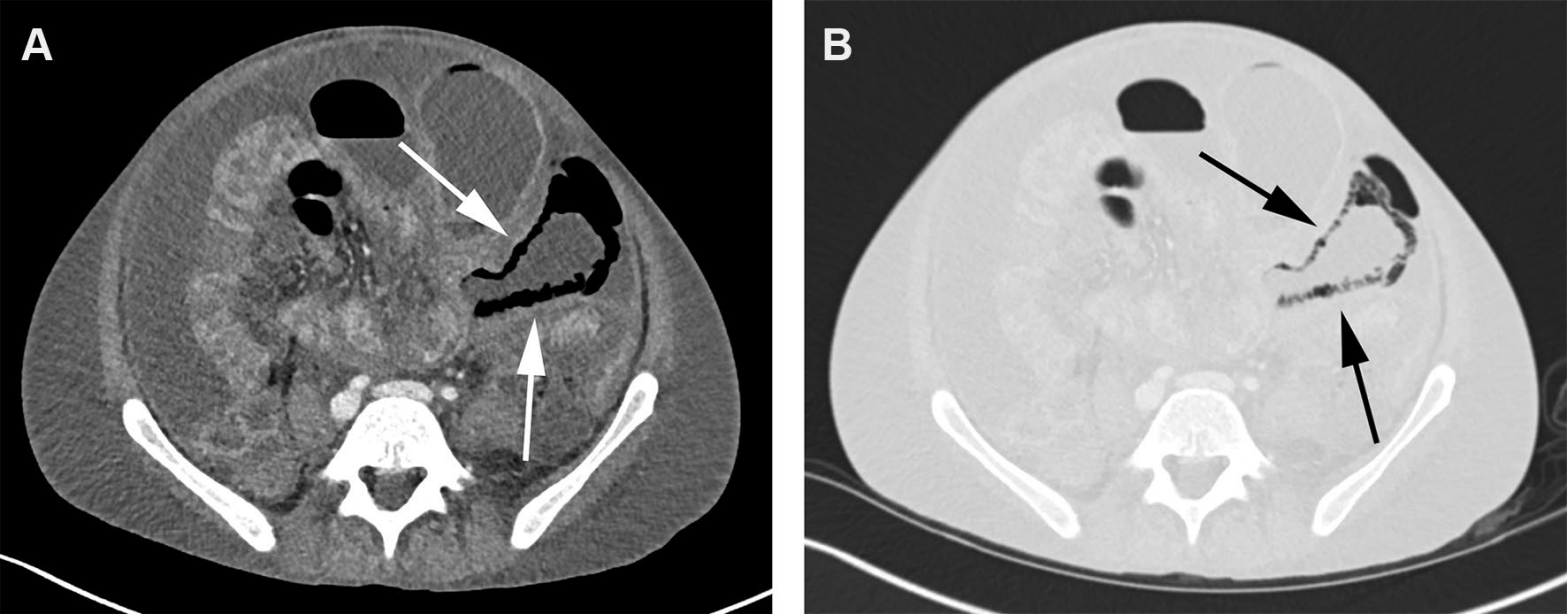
CT in the soft tissue (a) and lung window (b) of a 29-year-old male patient with PI in the small bowel (arrows) as well as gas in the mesenteric veins (not shown). The small bowel was distended and showed wall thickening as well. Ascites was present. The AST blood level was 18 IU/L (normal values 7–37 IU/L), the blood lactate level was 3.1 mmol/L (normal values 0.5–2.2 mmol/L). The patient recovered completely
